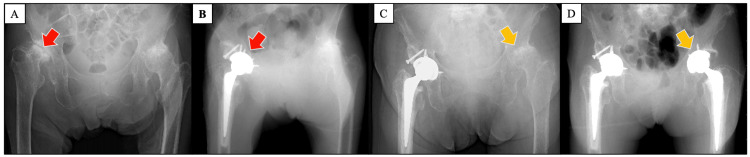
Pelvic X-ray transition (A) Pelvic X-ray at the time of admission to our hospital. (B) Two months after the initiation of TCZ; right THA was performed. Corresponding lesions are indicated by red arrows in figures (A) and (B), respectively. (C) One year after TCZ was initiated; left hip joint destruction had progressed. (D) Two years after the initiation of TCZ; left THA was performed. The corresponding lesions are marked with yellow arrows in figures (C) and (D), respectively. TCZ, tocilizumab; THA, total hip arthroplasty
